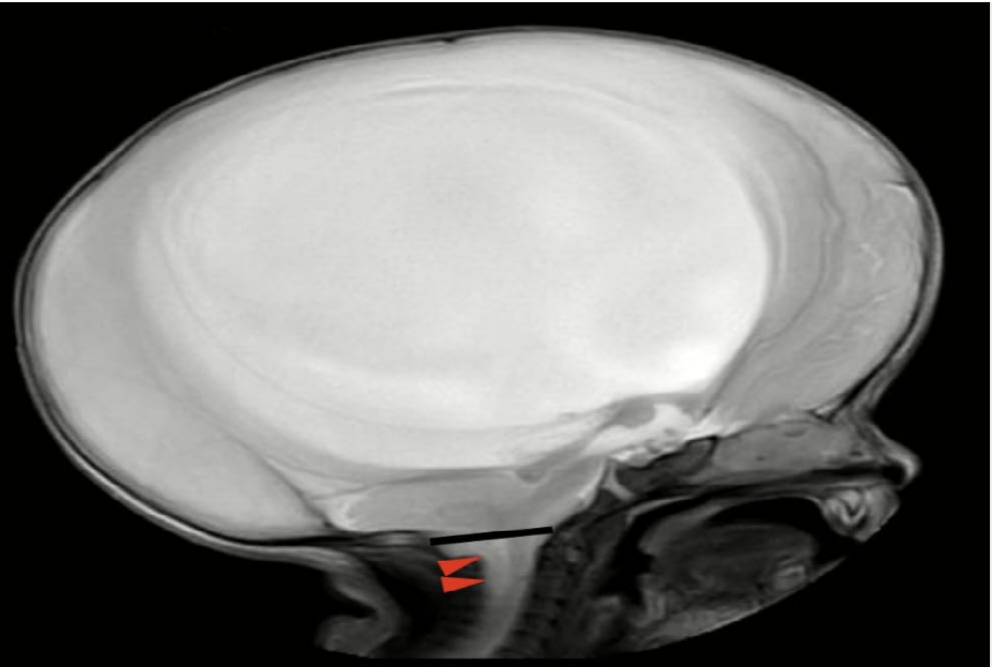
Representative sagittal T2‐weighted MRI of the brain shows a small, crowded posterior fossa with descent of the cerebellar tonsils (red arrowheads) below the basion–opisthion line (black line).
